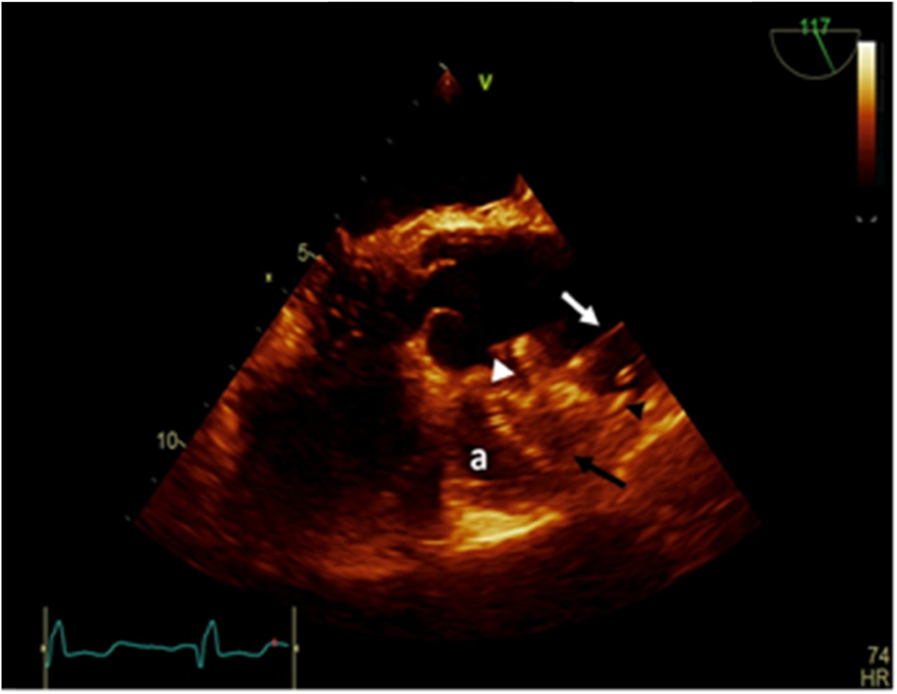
Inra-opperative Trans-esophageal echocardiography. Completed transcatheter closure of pseudoaneurysm neck with Amplatzer ASD occluder device 18 before it. A pseudoaneurysm. White arrow head: pseudoaneurysm neck Black arrow head: RA disk. Black arrow: LA disk white arrow: device cable
